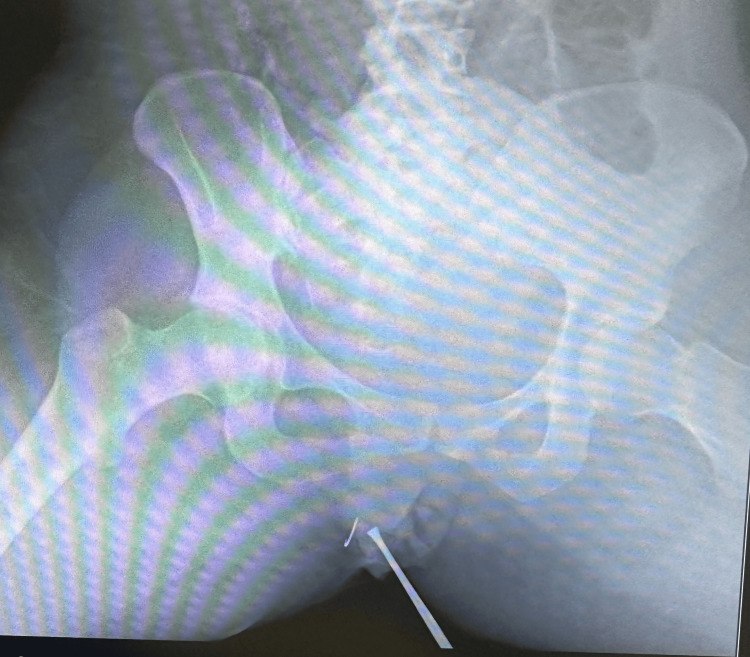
Radiographic localization with surface marking. The suspected target area was initially marked on the skin surface using a surgical instrument.
